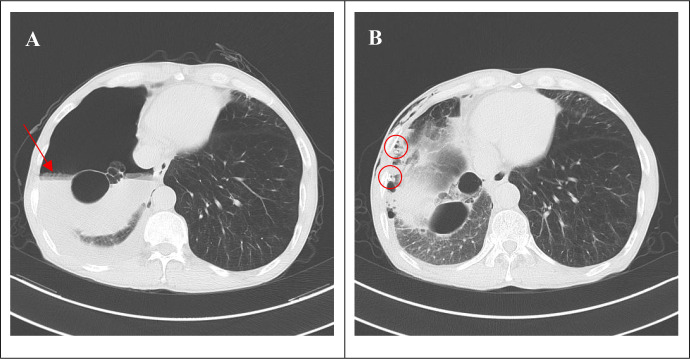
Imaging of hydropneumothorax. (A) Chest CT on June 18, 2022 (before treatment for hydropneumothorax): right-sided pneumothorax, pleural effusion, and an air-fluid level (arrow). (B) Chest CT on June 21, 2022 (after treatment for hydropneumothorax): two chest drainage tubes in place (circle), showing near-complete resolution of pleural gas and fluid, with good lung re-expansion.
